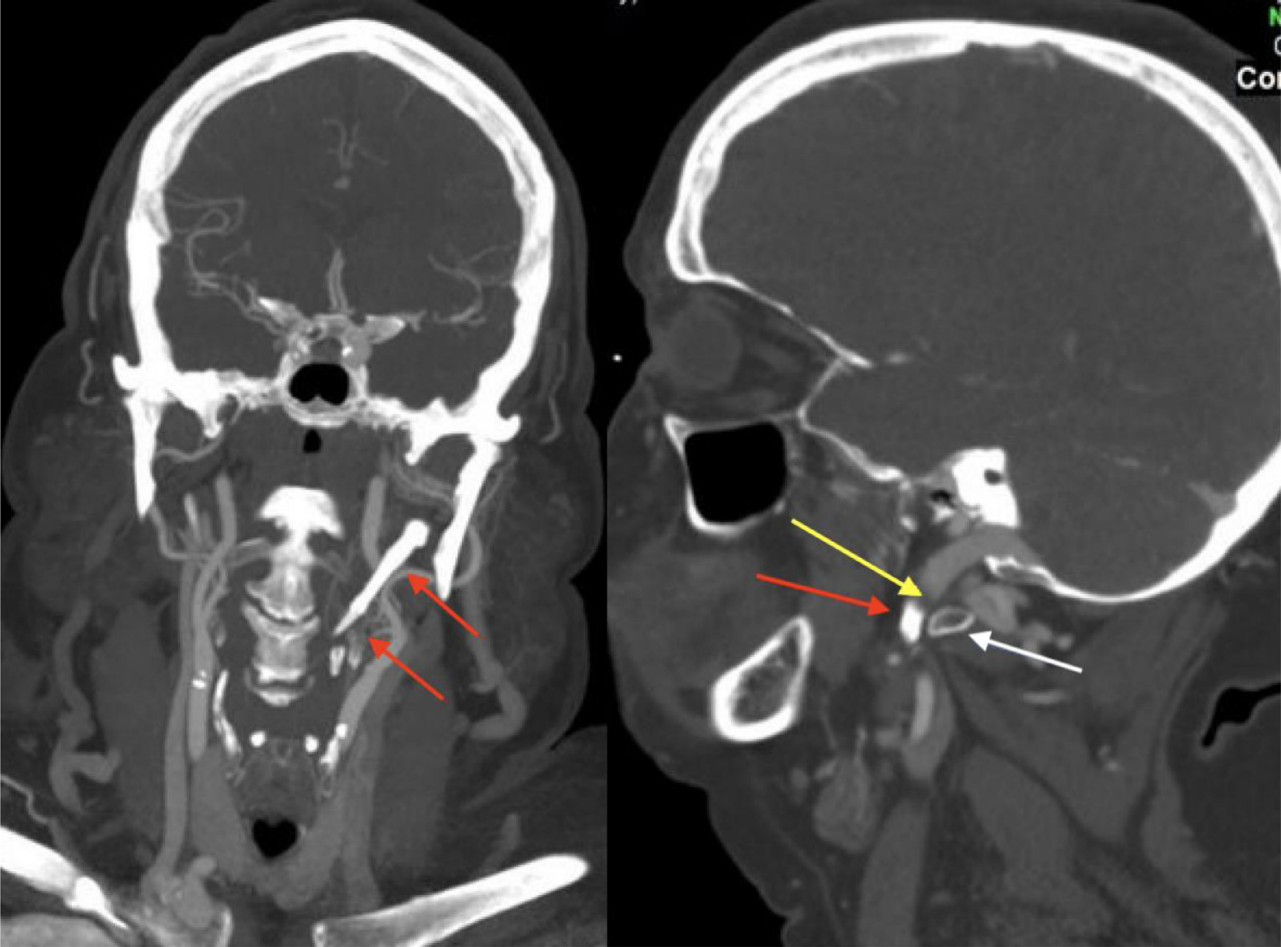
(A) (left) CTA coronal section, (B) (right), CTA sagittal section: demonstrating a markedly calcified and enlarged stylohoid ligament (yellow arrows) seen in the left neck and abuts the transverse process of C1 (white arrow). The left internal jugular vein (yellow arrow) is severely compressed by these two structures impeding flow.
